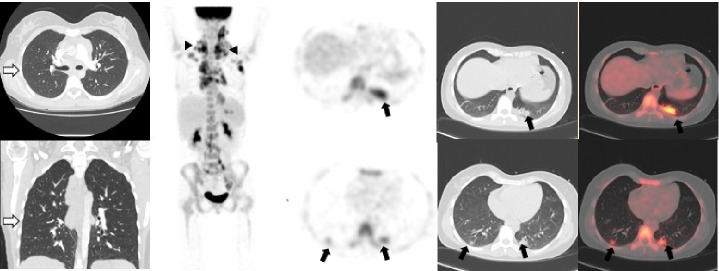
Angiotomografía de tórax previa a la PET/CT en la que no hubo evidencia de compromiso parenquimatoso (flechas blancas). En la PET/CT F18-FDG se encontraron múltiples ganglios hipermetabólicos cervicales, supraclaviculares, axilares bilaterales y mediastinales con SUVmáx de 10,7, relacionados con la enfermedad de base (cabezas de flechas). En el tórax (flechas negras) se observaron opacidades parenquimatosas con patrón de vidrio esmerilado localizadas en ambos segmentos posteriores de los lóbulos inferiores, pero con mayor compromiso del lado izquierdo, con aumento de la actividad metabólica y SUVmáx de 5,6.
